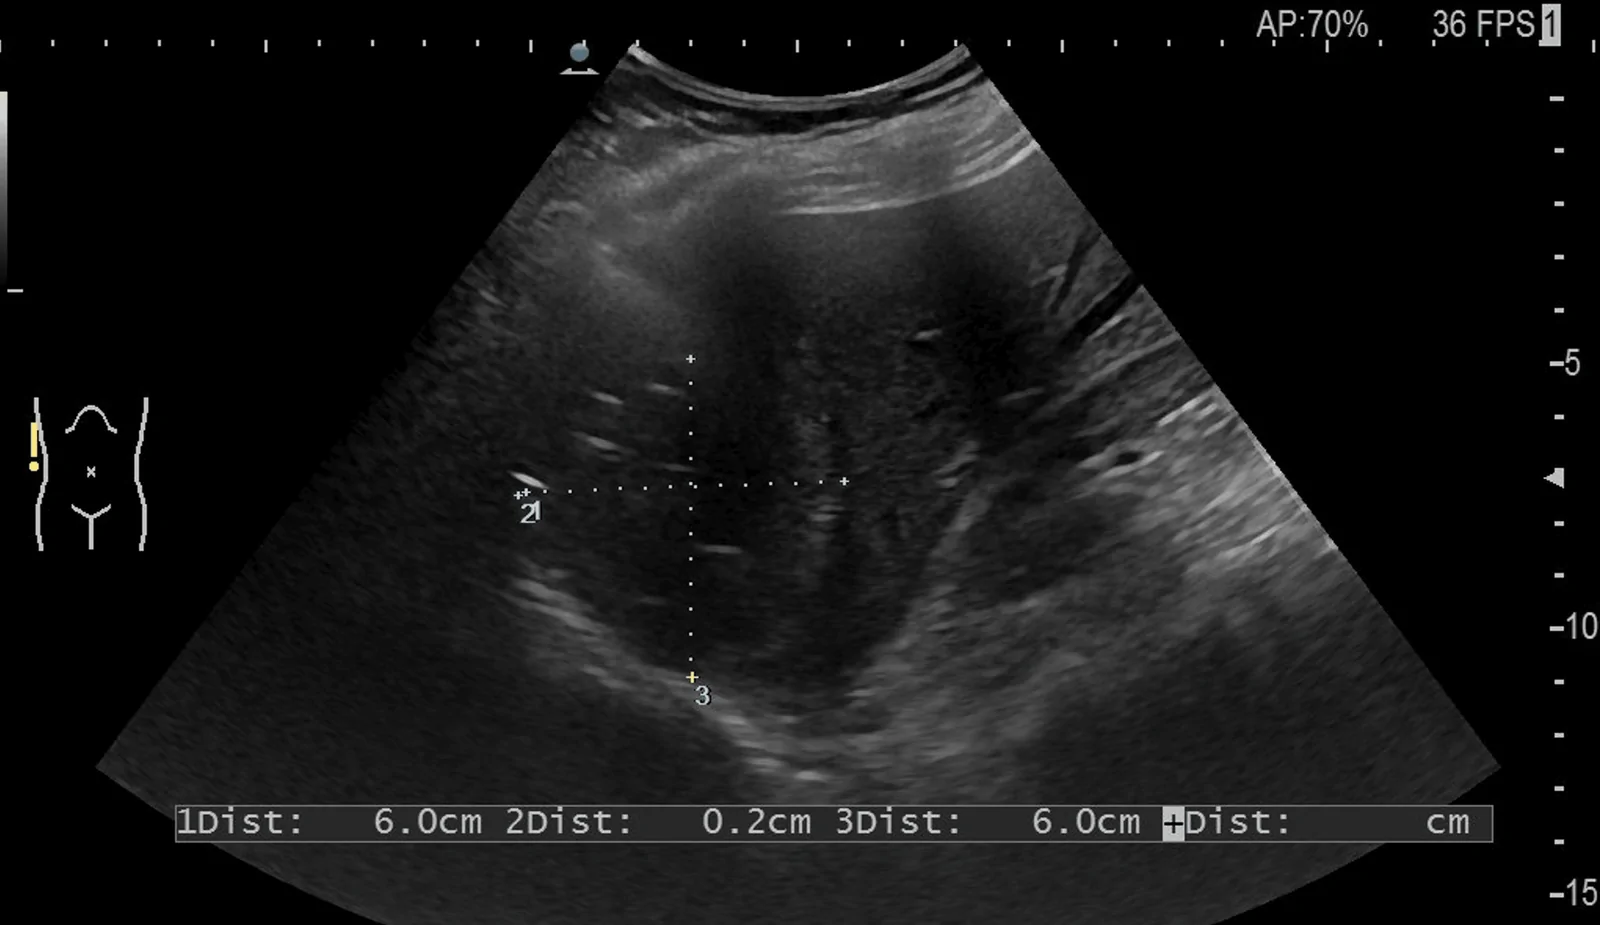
Initial ultrasonography of the right hepatic lobe showing a well-defined, predominantly hypoechoic lesion measuring approximately 60 × 55 × 60 mm, with mixed internal echoes and a peripheral rim The lesion was initially interpreted as a hepatic abscess.
